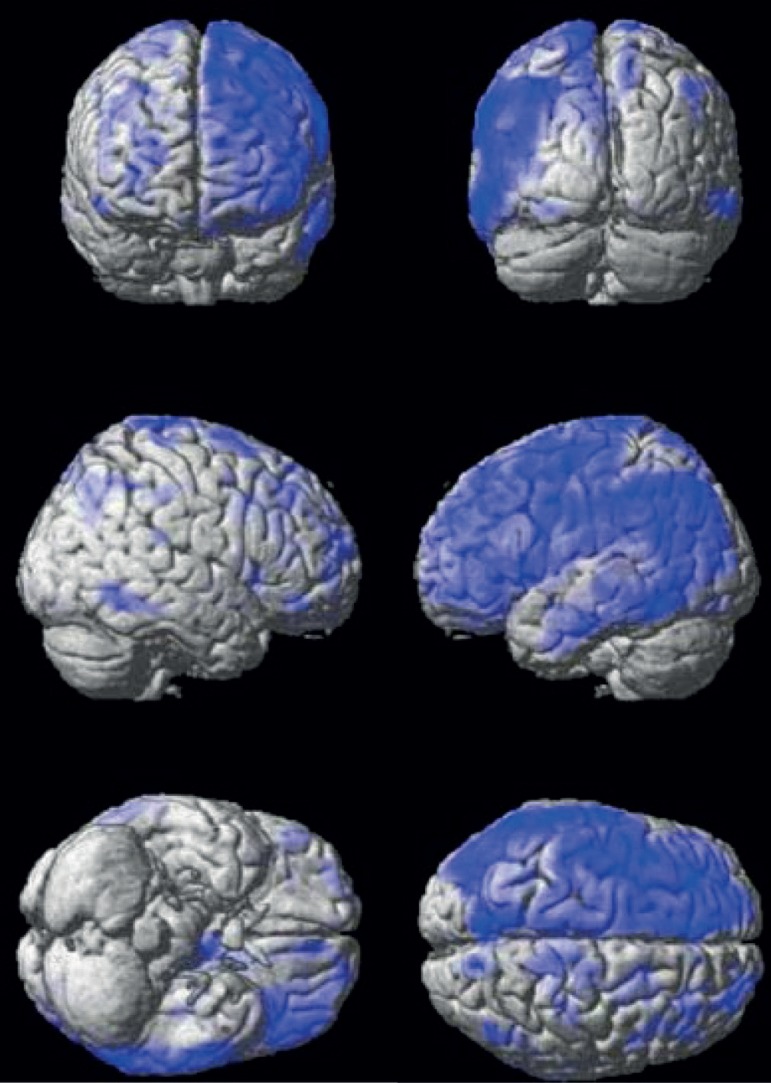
^18^F-FDG PET 3D images (in order from top to bottom:
